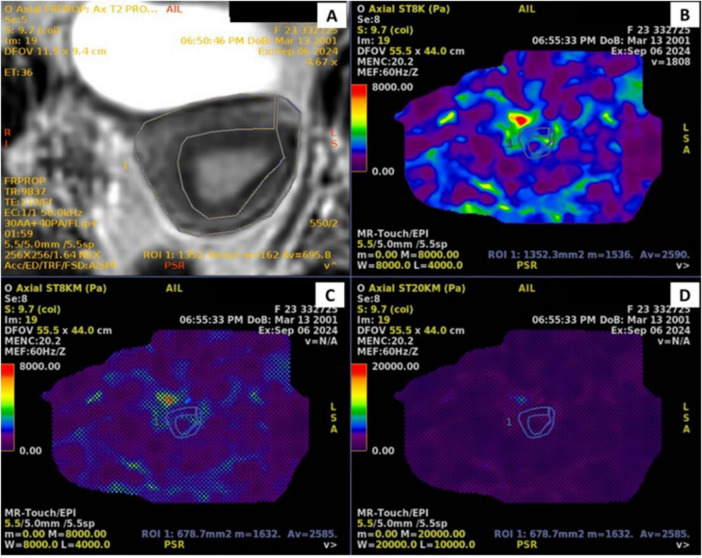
Pelvic MRI of a 30-year-old patient. (A) Axial T2 SE. (B) Elastography color map. (C) Elastography wave map. (D) Stiffness map.
